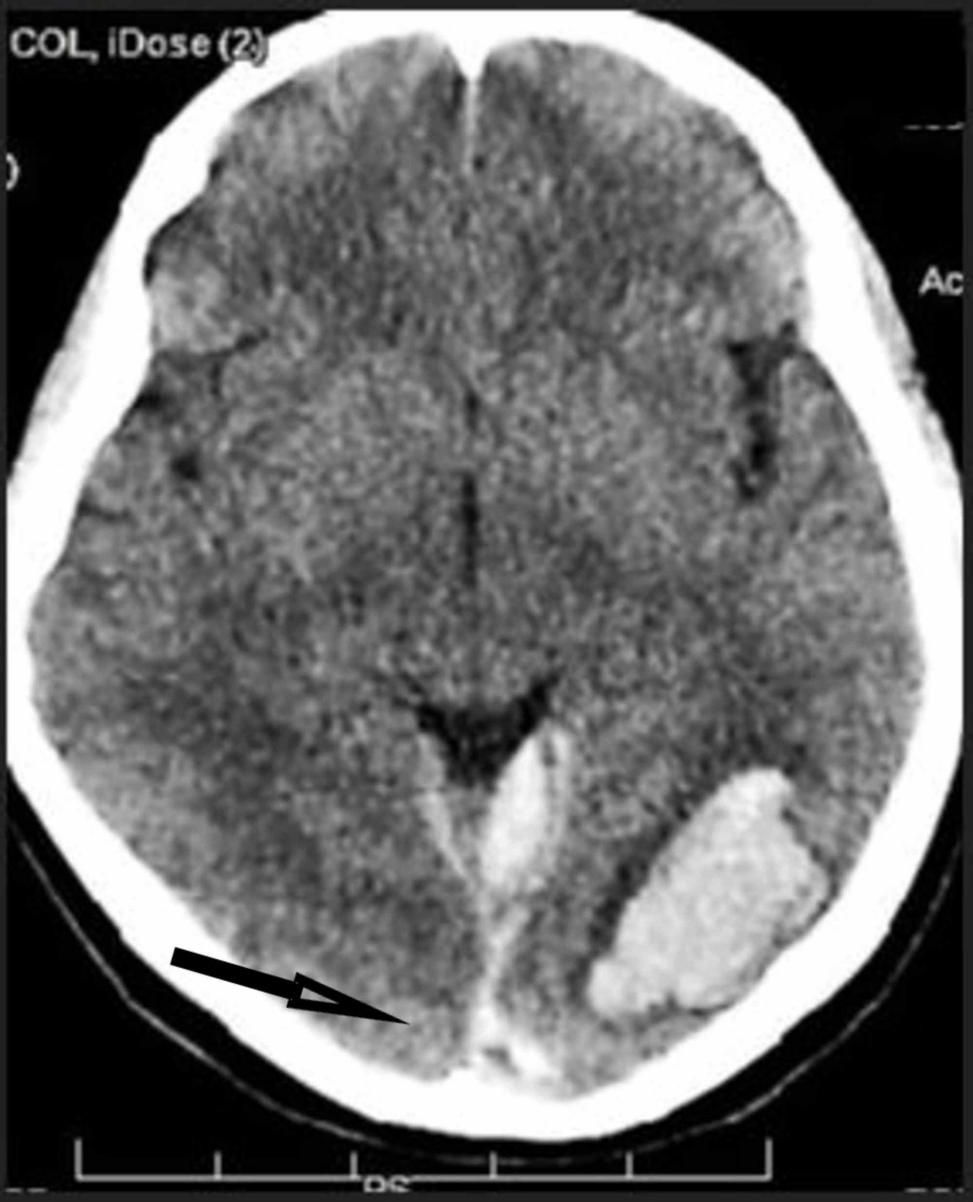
Computed tomography without contrast showing left occipital lobe intracerebral hemorrhage, with subdural extension (arrow)
